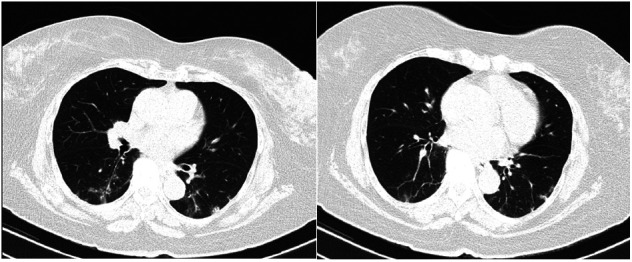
Seventy-seven-year-old female with a 5-day history of cough with fever and headaches. CT scan of the chest showing bilateral subpleural mixed ground-glass opacities GGO and consolidation with traction bronchiectasis (Negative initial PCR testing. Positive repeat PCR within 3 days)
